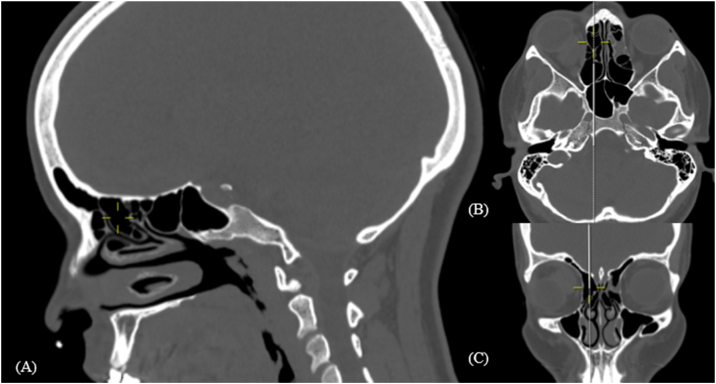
Supra bulla frontal cell. Computed Tomography: mark shows right supra bullar frontal cell in sargital (A), axial (B) and coronal (C) reconstructions.
